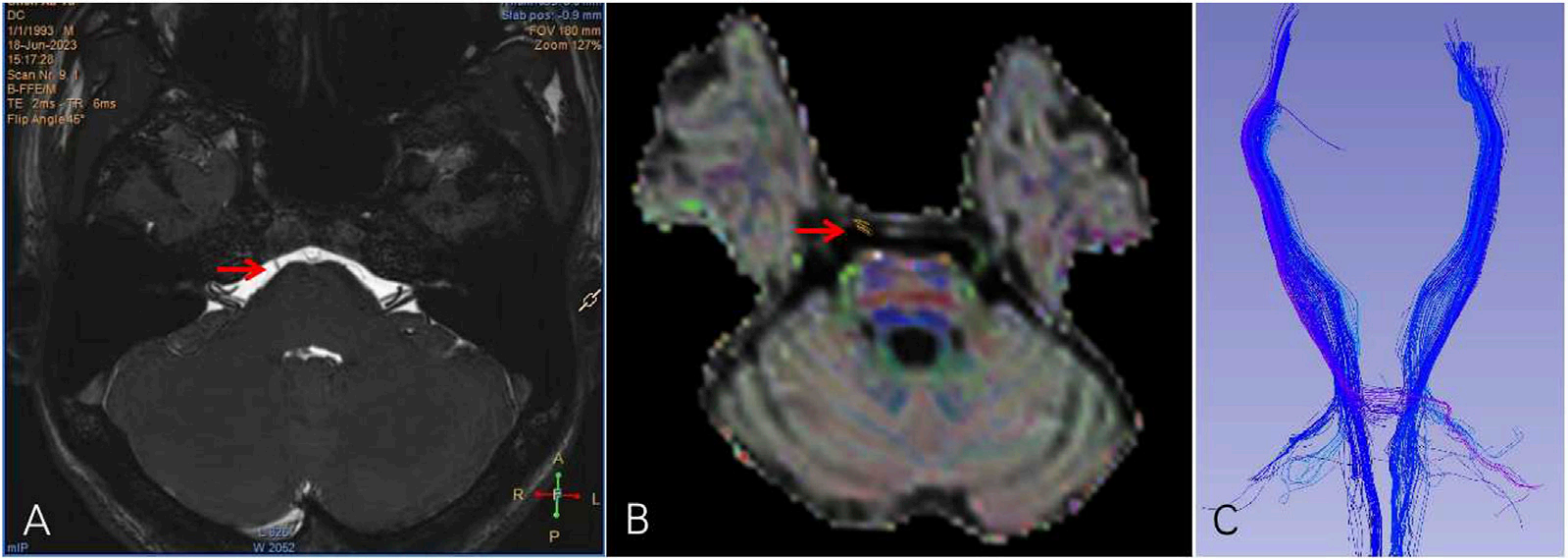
MRI and DTI images of a patient with DRS3. He had normal right abducens nerve [(A, B) arrow]. The left abducens nerve was absent, and the left corticospinal tract did not cross over to the opposite side (C).
